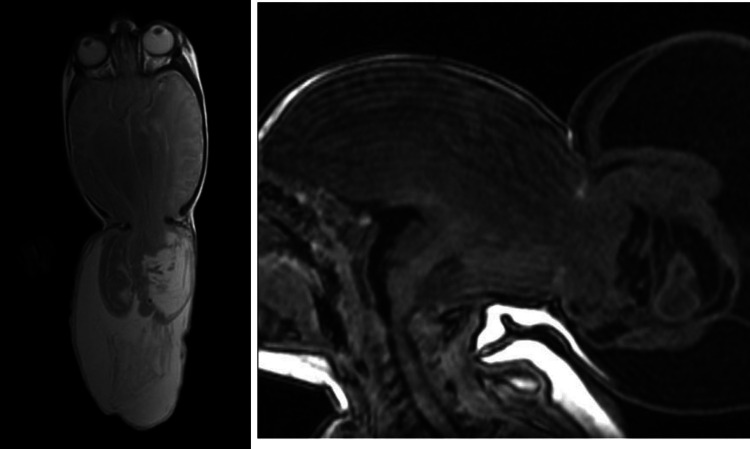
Preoperative postnatal MRI provides an axial and sagittal view of the preoperative MRI showing herniating neural tissue
